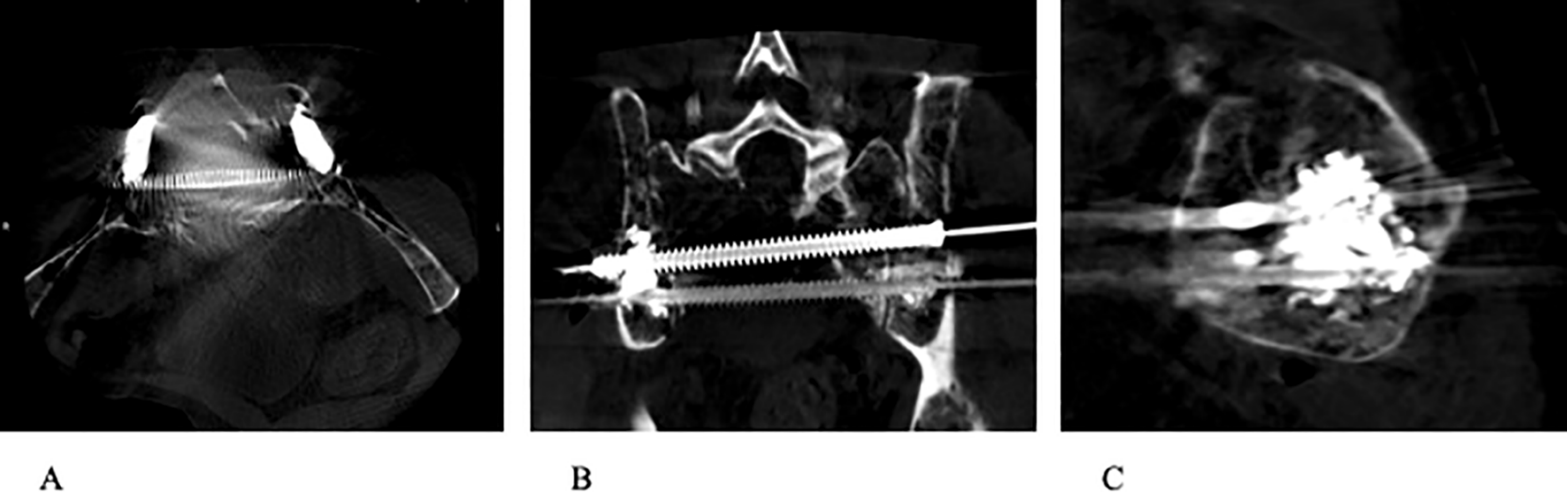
(A–C) Completion CT images (axial, coronal, and sagittal) demonstrating stabilization of sacrum with the use of percutaneous trans-sacral screw fixation and sacroplasty.
